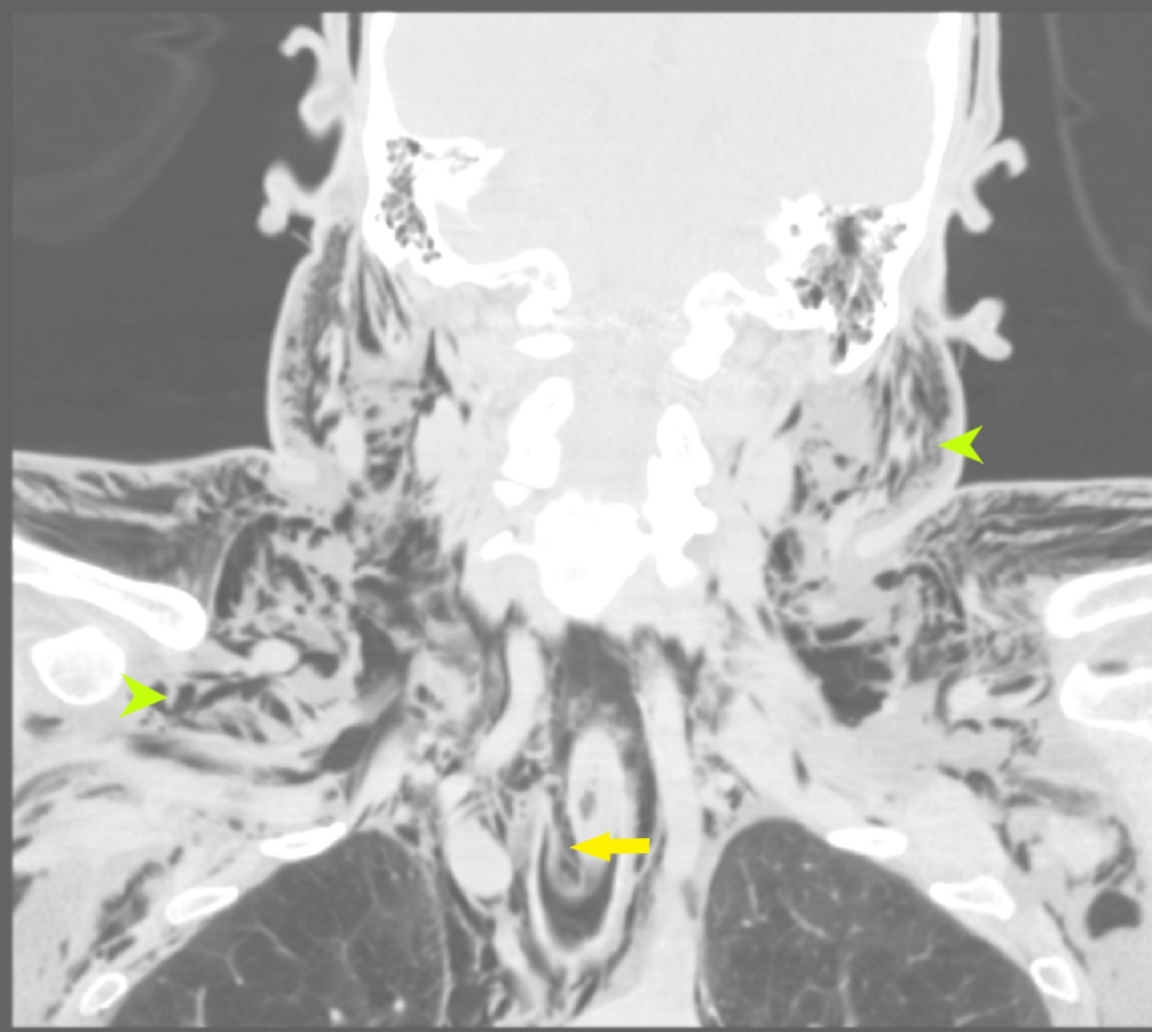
CT neck coronal section showing extensive subcutaneous emphysema (green arrowheads) involving the face, neck, and chest; narrowing of the oropharynx due to extensive air in the prevertebral space and retropharyngeal space is also present (yellow arrow) CT: computed tomography
